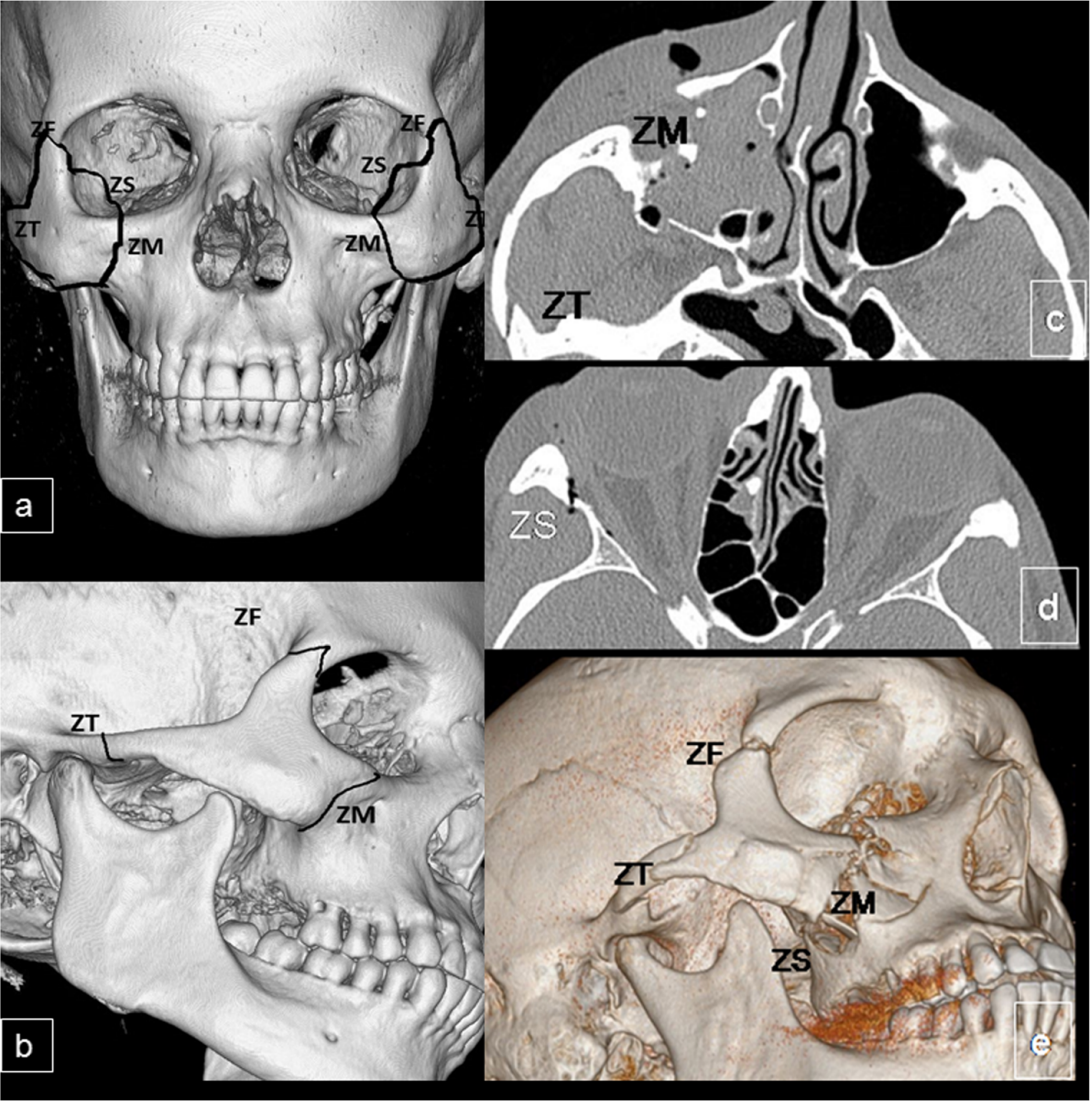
Zygomaticomaxillary complex. a, b Anatomy: three-dimensional CT image in frontal and lateral orientations shows the osseous anatomy of the zygomaticomaxillary complex: the zygomaticofrontal (ZF), zygomaticosphenoid (ZS), zygomaticomaxillary (ZM), and zygomaticotemporal (ZT) sutures. c–e Fracture of the zygomaticomaxillary complex. c, d Axial CT images show a nondisplaced fracture of zygomaticotemporal suture (ZT), comminution and angulation through the left zygomaticosphenoid (ZS), and significant displacement of the zygomaticomaxillary suture (ZM). e Three-dimensional CT images of the upper left facial region of the left ZMC fracture
